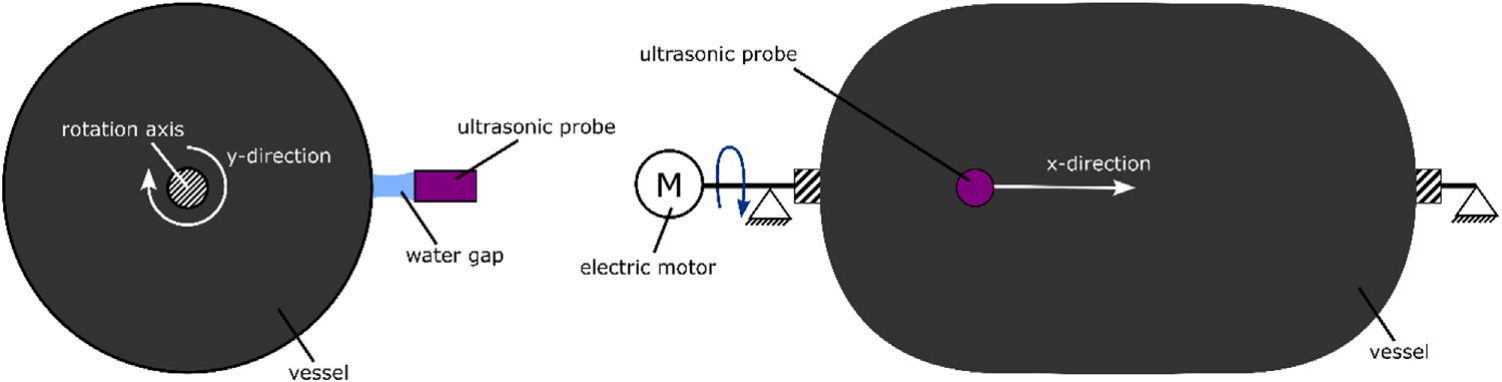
Test setup for ultrasonic inspection.
